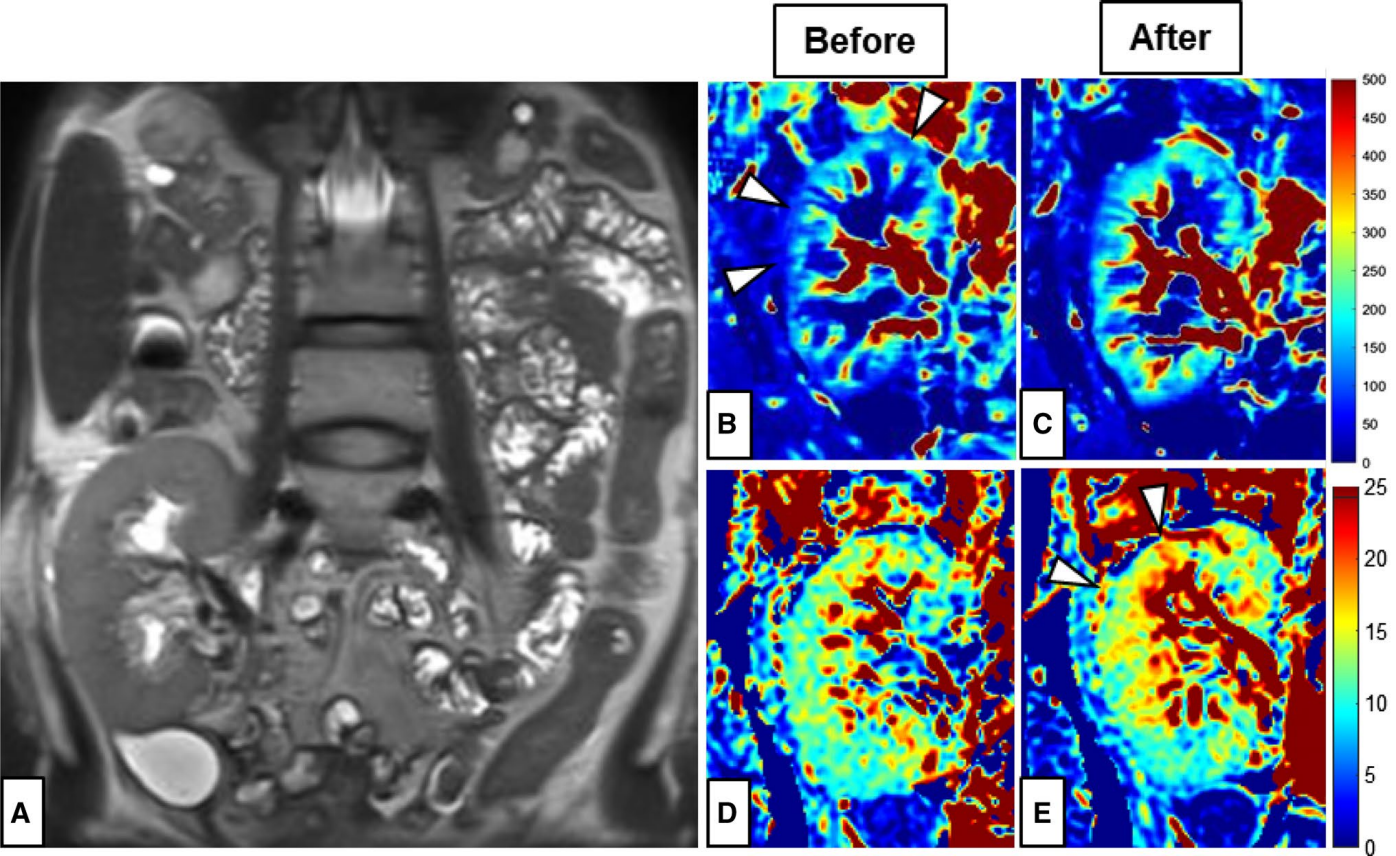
Functional MRI of the transplant kidney in the right iliac fossa: A Anatomical T2-weighted MR image depicting the renal artery (red arrow); RBF-maps in ml/min/100 g calculated from the ASL MRI before (B) and after (C) embolization; Perfusion fraction (PF) in % before (D) and after (E) embolization. Differences between measurements before and after embolization are highlighted by the white arrows pointing to the cortical perfusion deficit in the RBF map (B) and the improved cortical perfusion in the PF map in (E)
